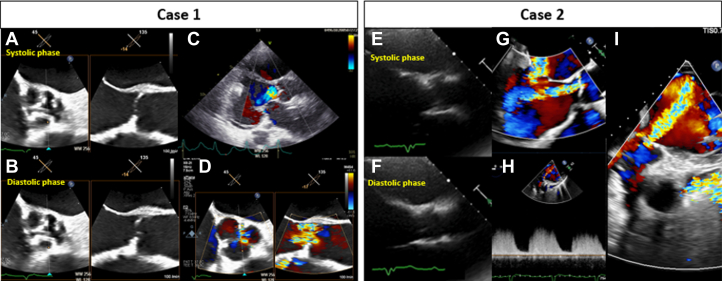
Baseline Echocardiographic Images for Cases 1 and 2 Case 1: (A and B) The aortic valve is shown in systole and diastole, respectively, demonstrating absent leaflet motion with a central coaptation defect. (C and D) Severe central aortic regurgitation is demonstrated on color Doppler imaging. Case 2: (E and F) The aortic valve is shown in systole and diastole, respectively, demonstrating preserved leaflet motion. (G and I) severe central aortic regurgitation is demonstrated on color Doppler imaging. (H) Continuous-wave Doppler demonstrates regurgitant flow extending beyond diastole and occupying a substantial portion of the cardiac cycle.
